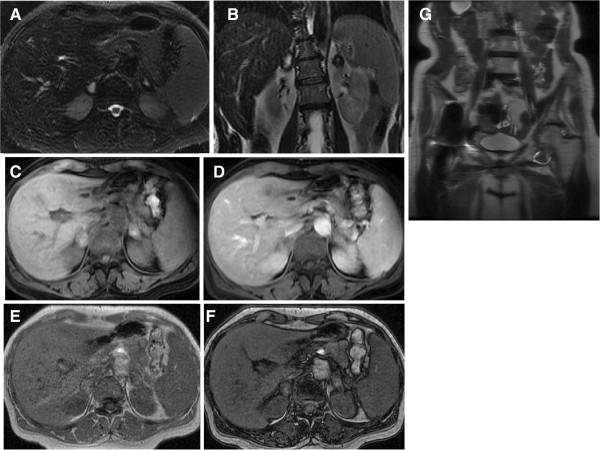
Abdominal magnetic resonance imaging obtained 15 days after surgery. Axial (A) T2 weighted image with fat suppression, coronal (B) T2 weighted image, in and out of phase axial (C, D) T1 images, axial (E, F) T1 weighted images with fat saturation before and after contrast administration, coronal (G) T2-weighted image. Bilateral adrenal lesions showing high signal both on T1-weighted (E) and on T2-weighted images (A, B), and low signal rim on T2-weighted images (A, B), representing haemosiderin. The lesions do not show any signal drop-off on T1 in- (C) and out- of phase images (D). After intravenous contrast administration (F) no enhancement is depicted. These findings are consistent with chronic adrenal hematomas. Note the signal void in the right pelvis due to the presence of a metallic right hip prosthesis.
